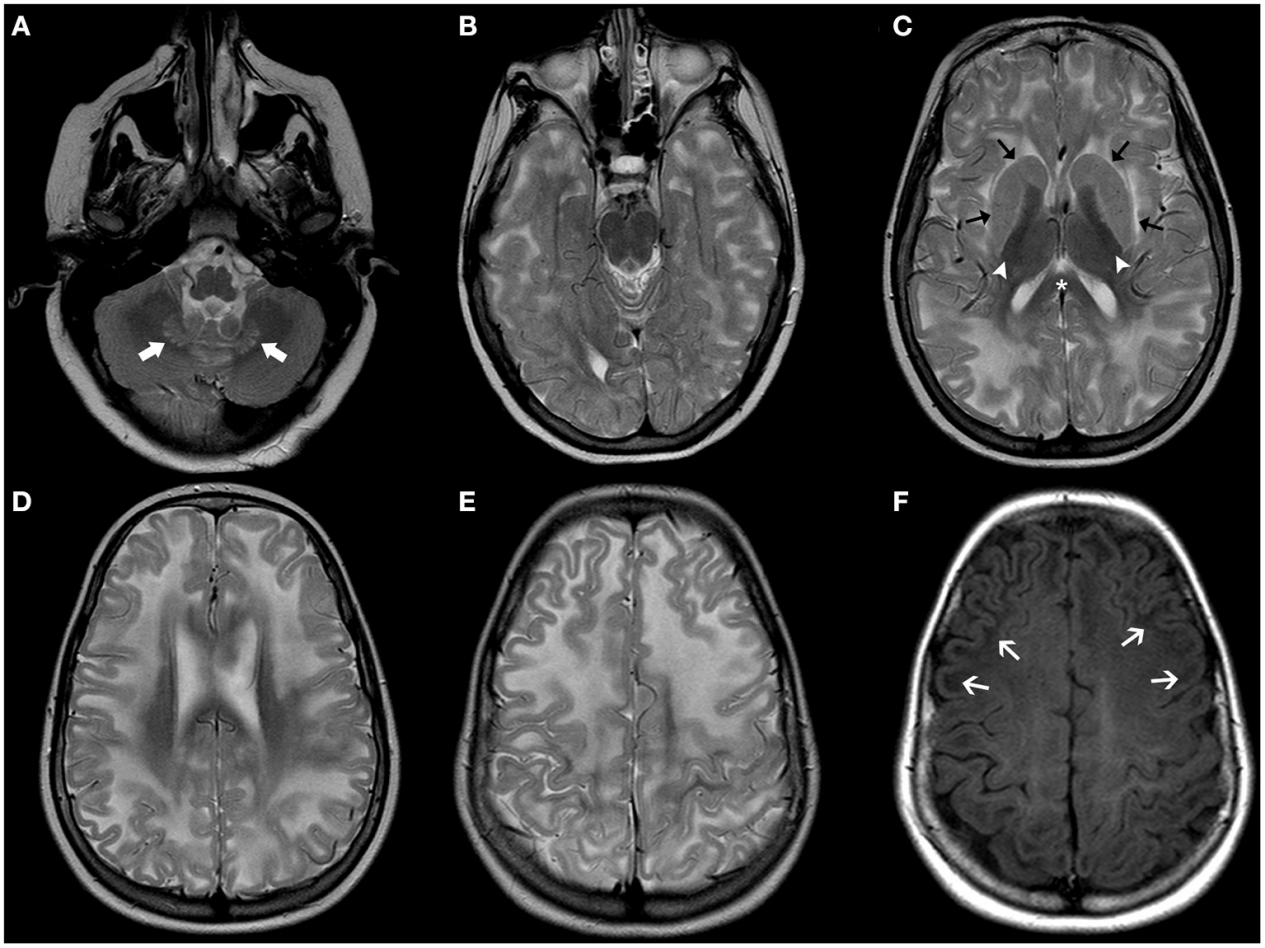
Brain MRI findings in L2-Hydroxyglutaric Aciduria. Axial T2WI (A–E) of an affected 11-year-old boy show diffuse, bilateral, and symmetric hyperintensity involving mainly the subcortical and deep cerebral white-matter, while the periventricular white-matter, corpus callosum (asterisks), and internal capsules (white arrowheads) are relatively spared. There is also mild, symmetric hyperintensity of the caudate nuclei and putamina (black arrows) and dentate nuclei (thick white arrows). Axial T1WI (F) demonstrate bilateral, symmetric hypointensity involving mainly the subcortical cerebral white matter/U fibers (white arrows).
